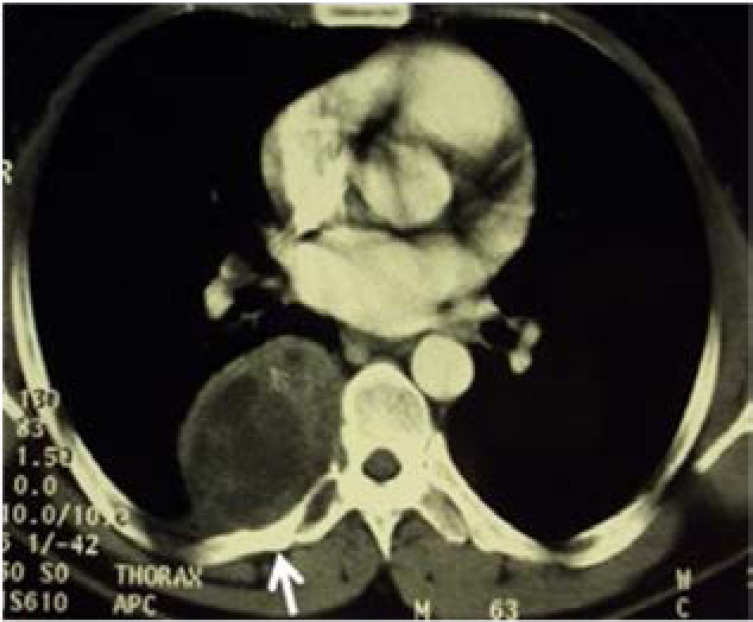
Transverse section thoracic CT, showing a multivesicular fluid lytic mass involving the transverse process and pedicle of the 7th dorsal vertebra and the posterior arch of the 7th rib (arrow).
